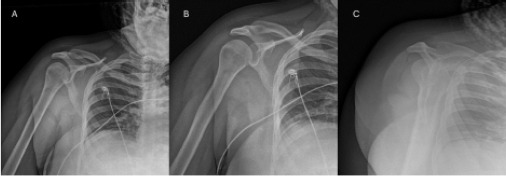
Three-view radiographs of the right shoulder demonstrating no fracture or dislocation in the A) anteroposterior externally rotated, B) anteroposterior internally rotated, and C) scapular Y projections.
